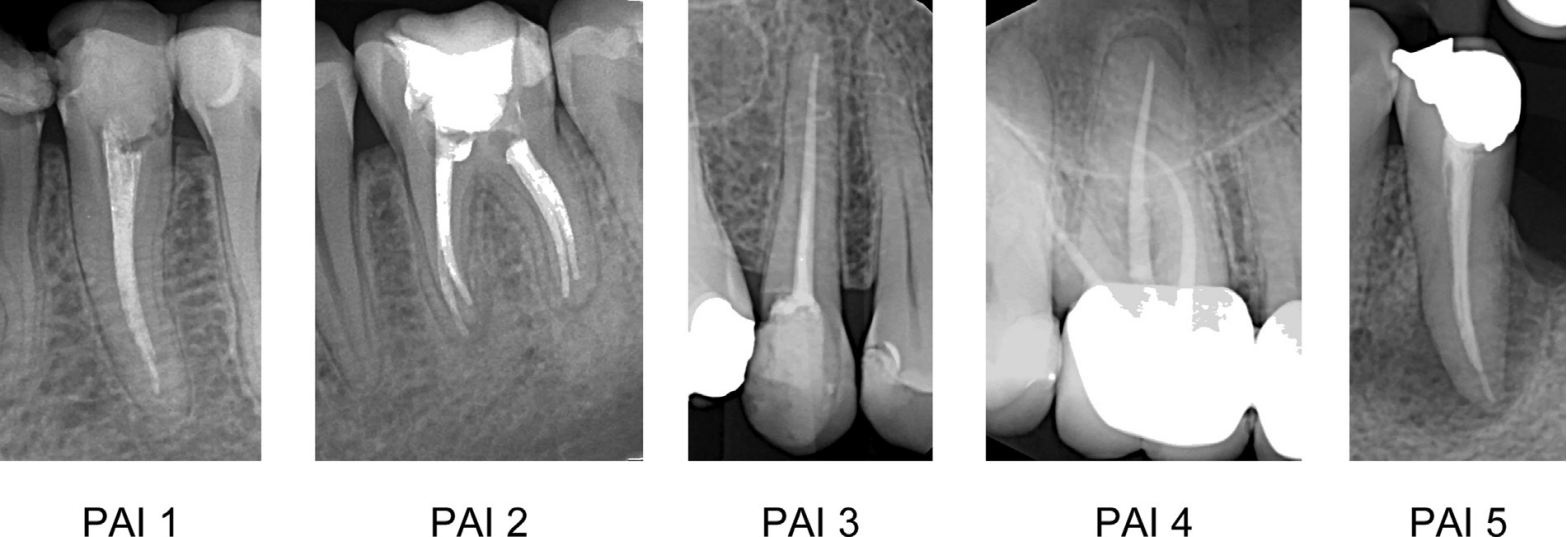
Periapical radiographs of endodontically treated teeth originating from investigated material representing each of the five periapical index (PAI) scores. Absence of apical periodontitis: PAI = 1 and PAI = 2. Presence of apical periodontitis: PAI = 3, PAI = 4, and PAI = 5.
